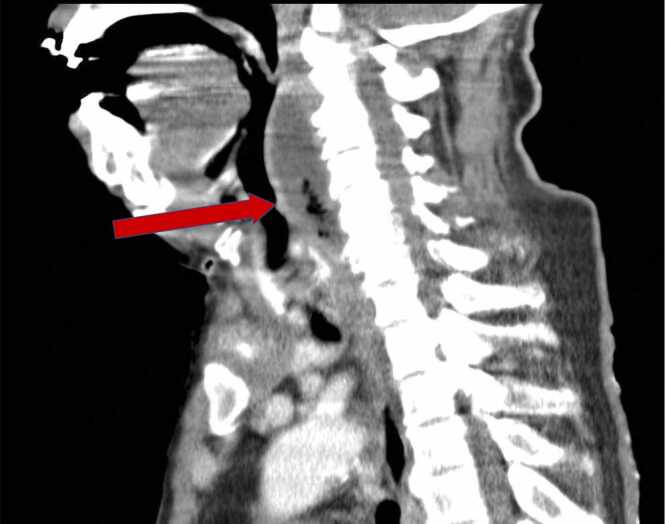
Sagittal view of an enhanced CT showing a region of fluid retention with gas in front of the cervical spine, with an appearance consistent with a retropharyngeal or prevertebral abscess (red arrow).
